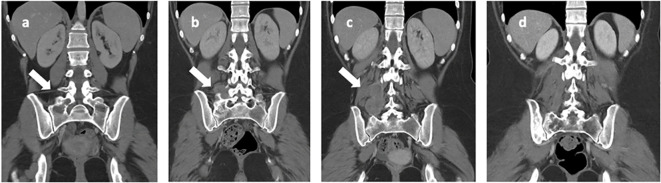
Iliac bone CT scans highlight: (A) a lesion with lytic features located in the right iliac wing and sacral wing (indicated by the arrow) at the onset of bone metastasis. In images (B, C), there is a progressive increase in the extension observed in the right paravertebral soft tissue (indicated by the arrows), as seen at the time of discontinuation of nivolumab. Image (D) depicts the nearly complete resolution observed at the last follow-up, which occurred 8 years after disease onset.
